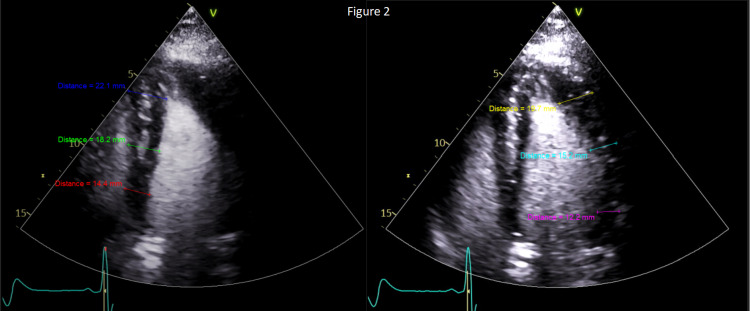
Echocardiogram with ventricular wall measurements and classic “ace of spades” shape. Left image: Transthoracic two-chamber view of the left ventricle and atrium, with free wall on the right side of the image. Contrasted with perflutren (lipid microsphere contrast agent). Right image: Transthoracic four-chamber view using perflutren. Left ventricle free wall to the right side of the image.
